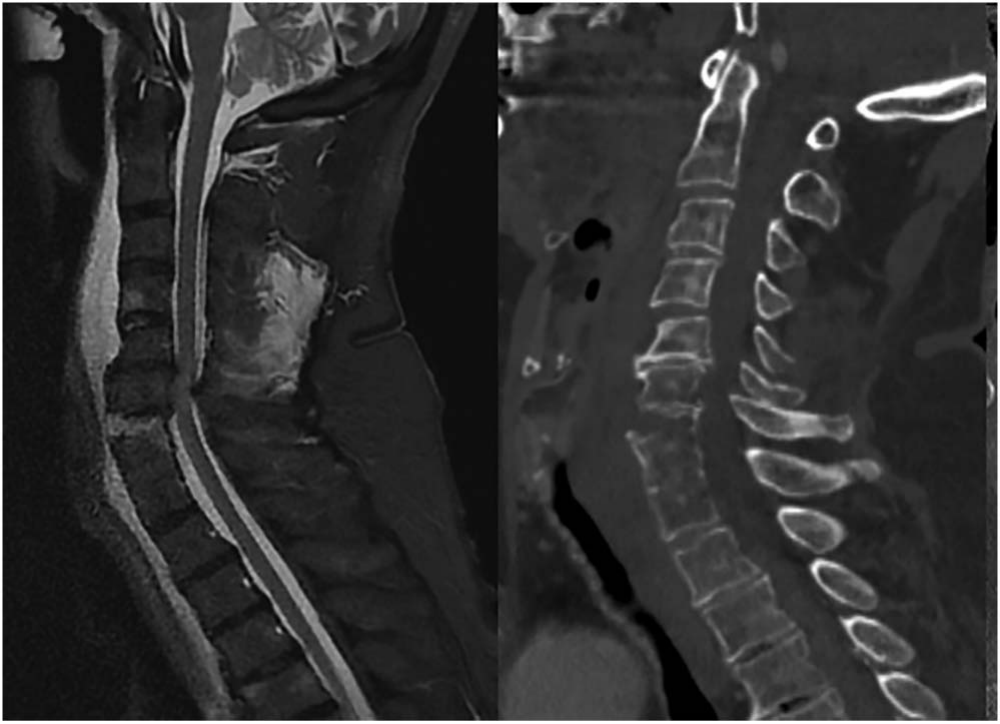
From the left, a cervical spine T2-w MRI and a bone CT, showing a C6-C7 fracture with canal stenosis and medullary compression.
